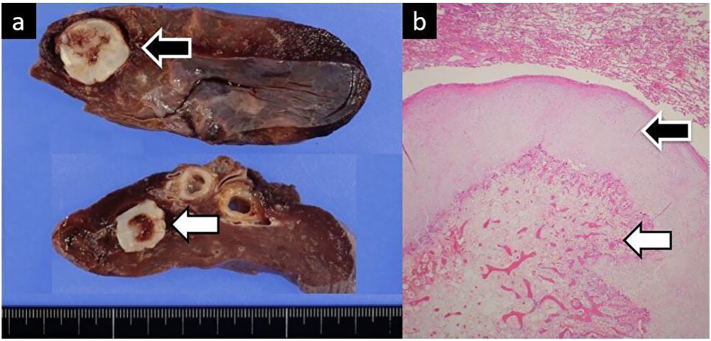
Computed tomography (CT) at initial presentation. a) CT shows a 1.4-cm nodule in the lower lobe of the left lung (black arrow). b) Positron emission tomography (PET) shows very little accumulation (white arrow).
